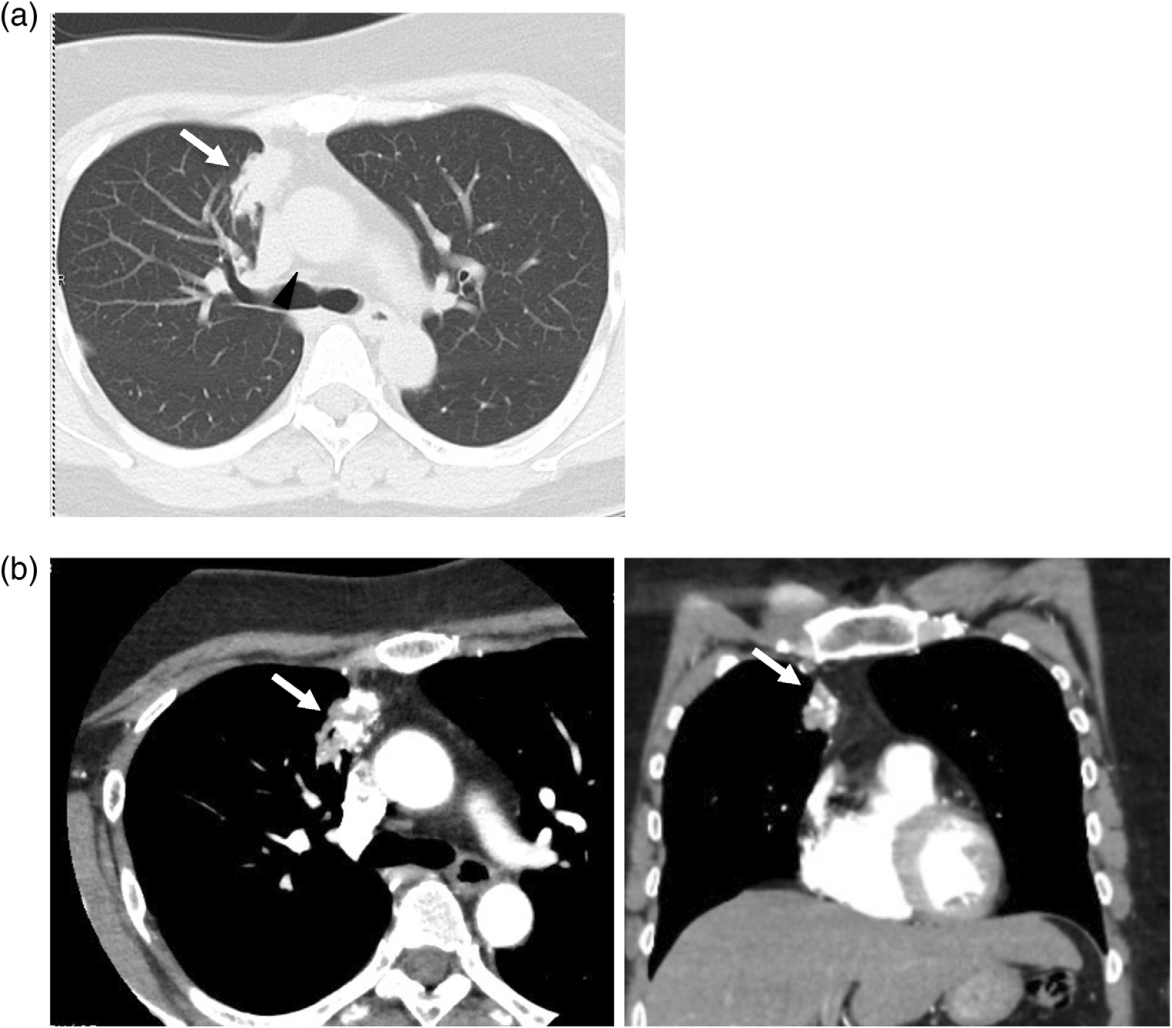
(a) Computed tomography (CT) showing a 31‐mm irregular nodule in the anterior segment (S3) of the upper lobe of the right lung (white arrow). (b) Contrast‐enhanced CT showed a tubular structure (white arrow).
